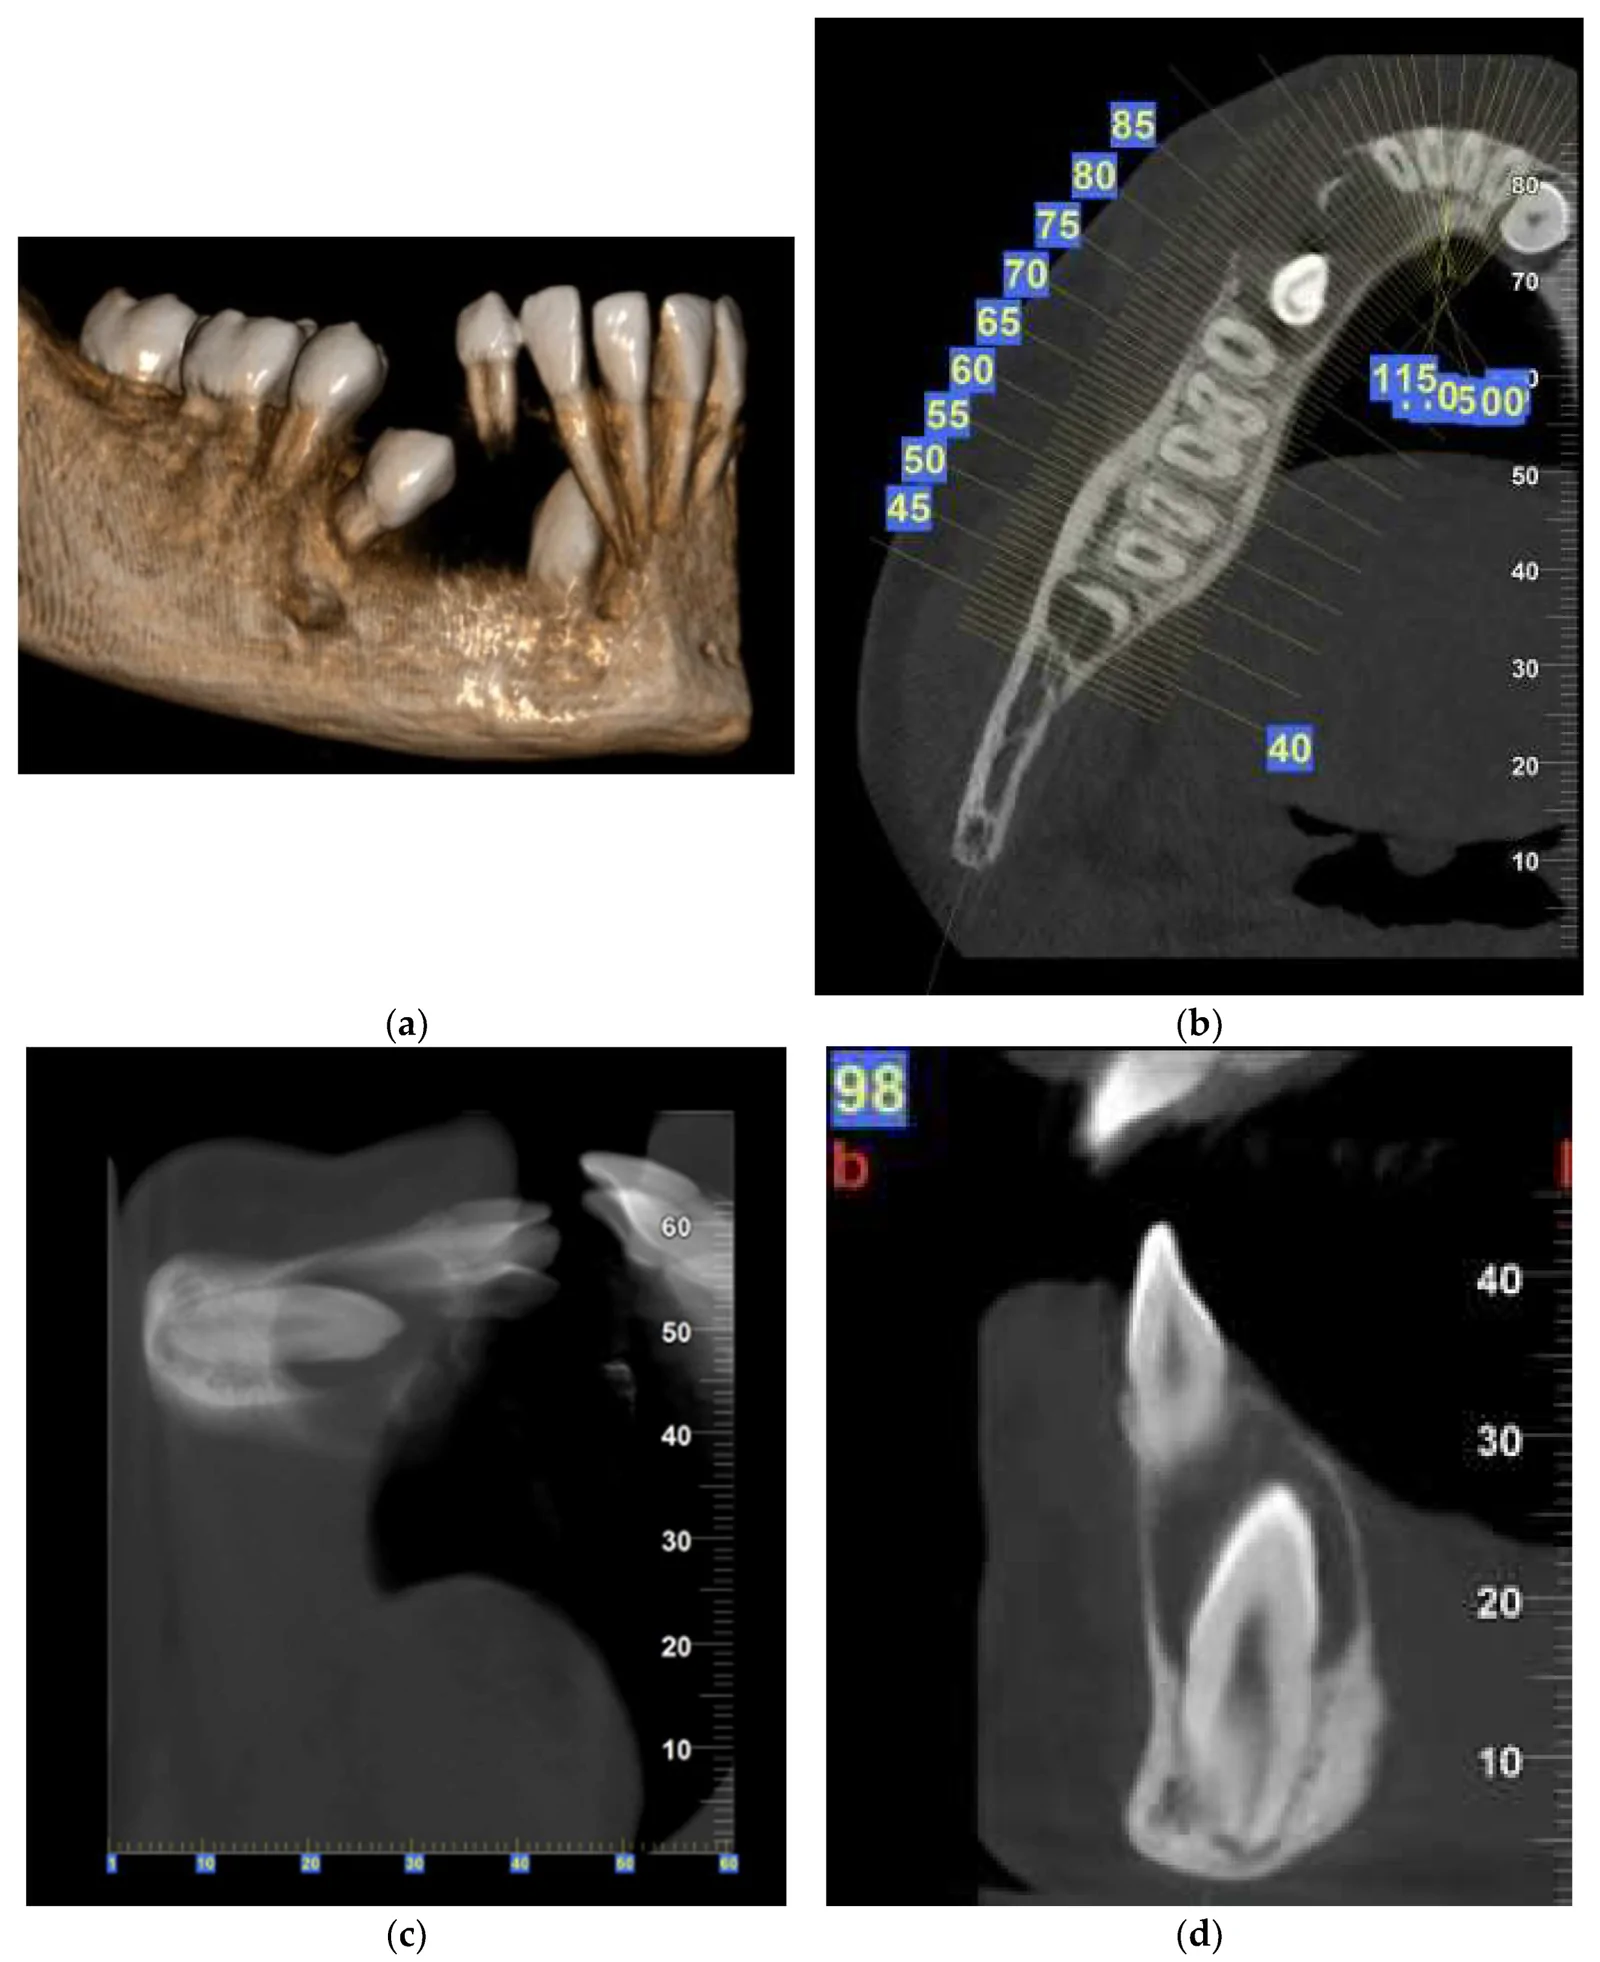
Initial CBCT assessment of the mandibular lesion: (a) three-dimensional reconstruction demonstrating the overall extent of the lesion and relationship with the impacted teeth; (b–d) multiplanar CBCT sections showing the lesion dimensions and anatomical relationships. The cyst measured approximately 30 × 25 × 20 mm (AP × SI × BL).
